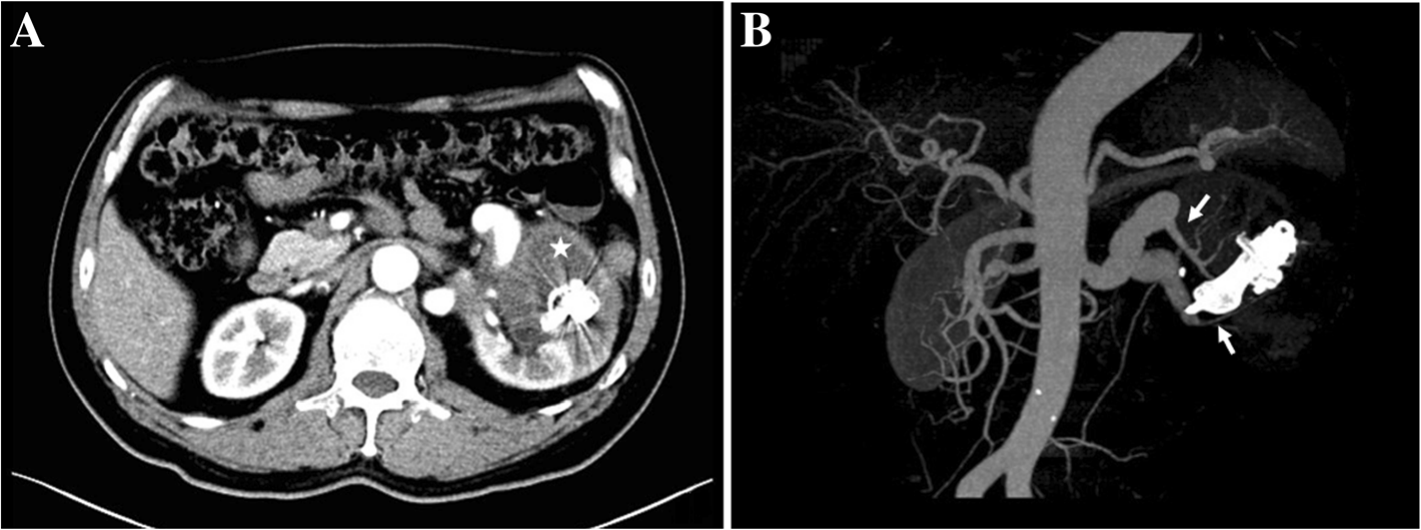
Repeat enhanced CT showed a thrombus developing in previously malformed blood vessels (white star). The residual left renal parenchyma displayed well (a). 3D CT reconstruction demonstrated that the normal branch of the renal artery was retained (white arrows) (b)
